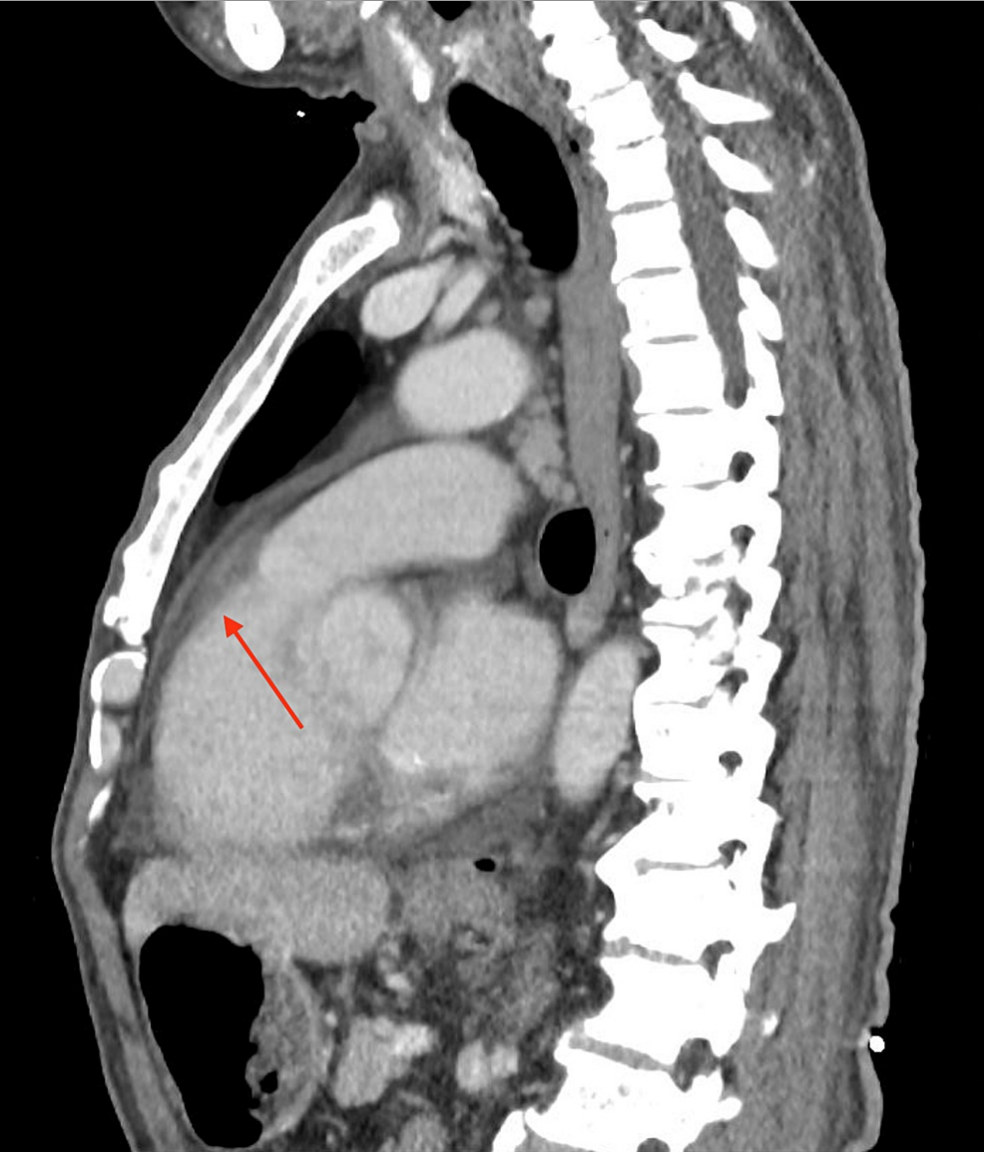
CT thorax displaying pericardial effusion (red arrow). CT: computed tomography.
